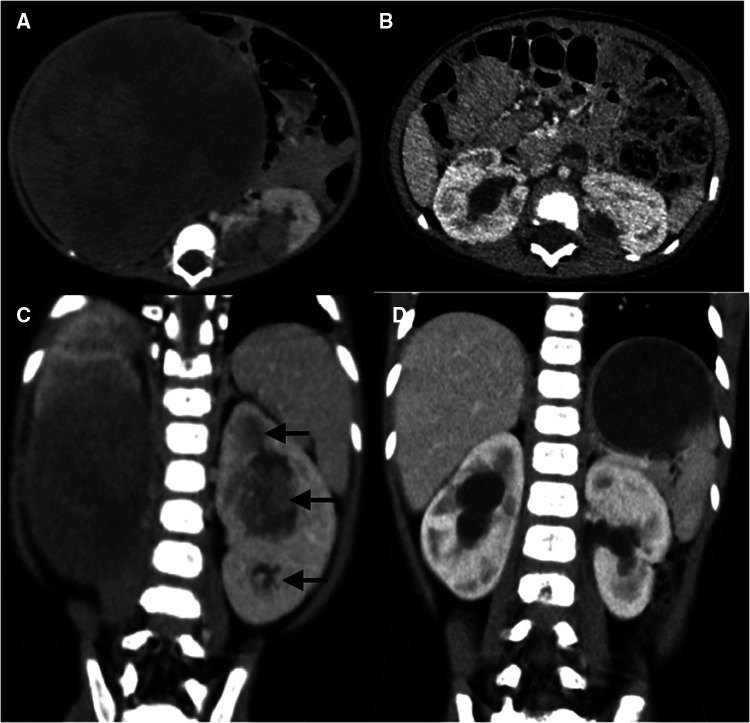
Abdominal CT scans of case 6. (A,C) Preoperative CT images reveal a huge renal mass in the right kidney and multifocal neoplasms in the left kidney (black arrow), with bilateral renal sinus invasion. (B,D) Postoperative CT images reveal multiple perfusion defects in both kidneys and bilateral mild hydronephrosis, without evidence of recurrence or metastasis.
